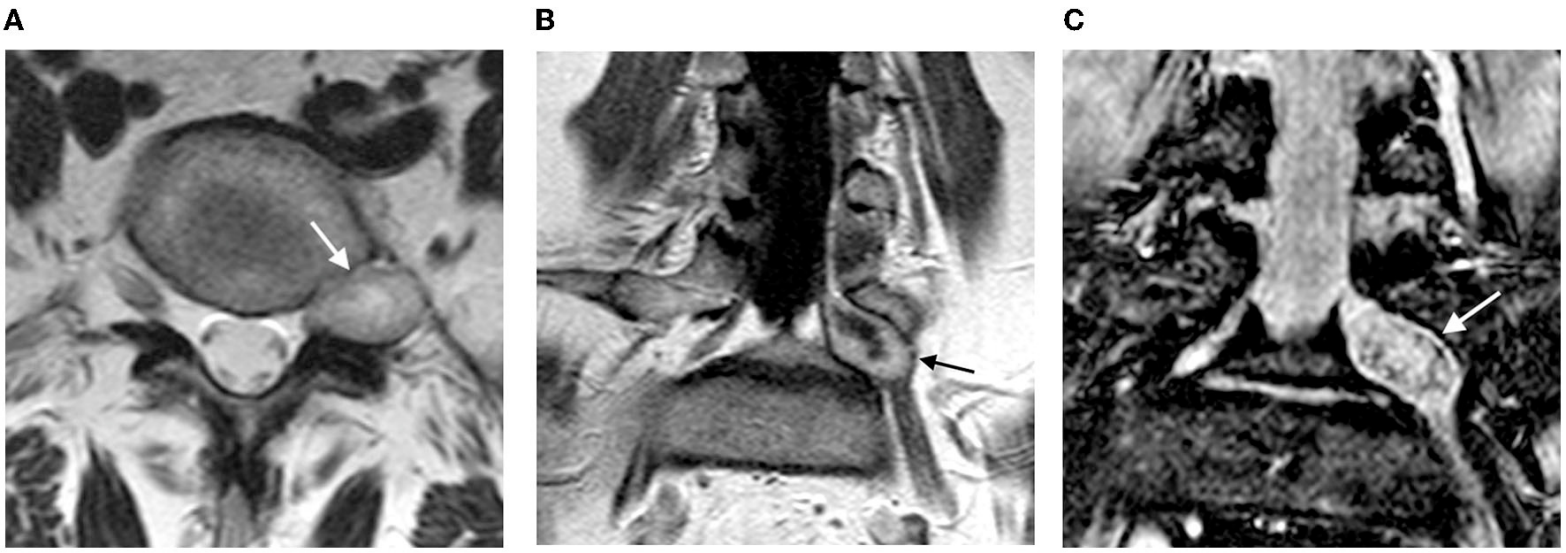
Magnetic resonance imaging of the lumbar spine: (A) axial view, T2-WI mode; (B) frontal view, T1-WI contrast mode; (C) frontal view, T1-FS post-contrast (arrow shows a cystic-solid tumor of the spinal nerve, size 3.2 × 1.5 × 1.5 cm; the tumor accumulates contrast).
